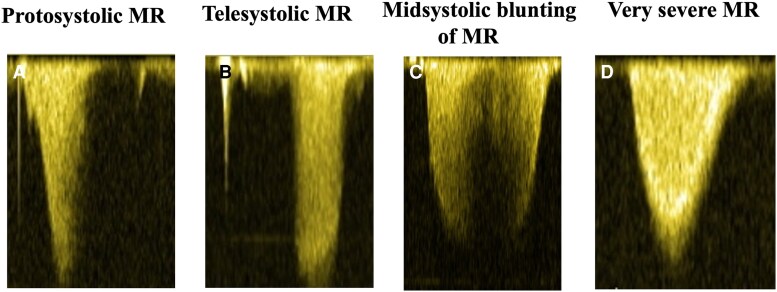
Timing and regurgitant duration in mitral regurgitation. (*A*) shows a protosystolic MR flow in a patient with atrial fibrillation and (*B*) a telesystolic signal in mild mitral valve prolapse with a centrally oriented jet. (*C*) In mild FMR, there is significant blunting of midsystolic regurgitation due to mitral valve closure. In this situation, tracing the entire envelope will yield a too large VTI and overestimation of RegVol when using the PISA method. In (*D*), a patient with very severe FMR is shown with a triangular and very intense Doppler signal with small VTI.
